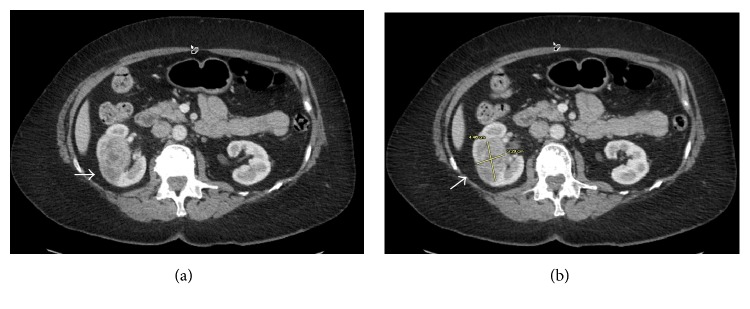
Computed tomogram of the abdomen-pelvis with contrast shows a 4.5 × 3.3 cm heterogeneous, enhancing soft-tissue density mass within the right kidney with a patent right renal vein (white arrow).
